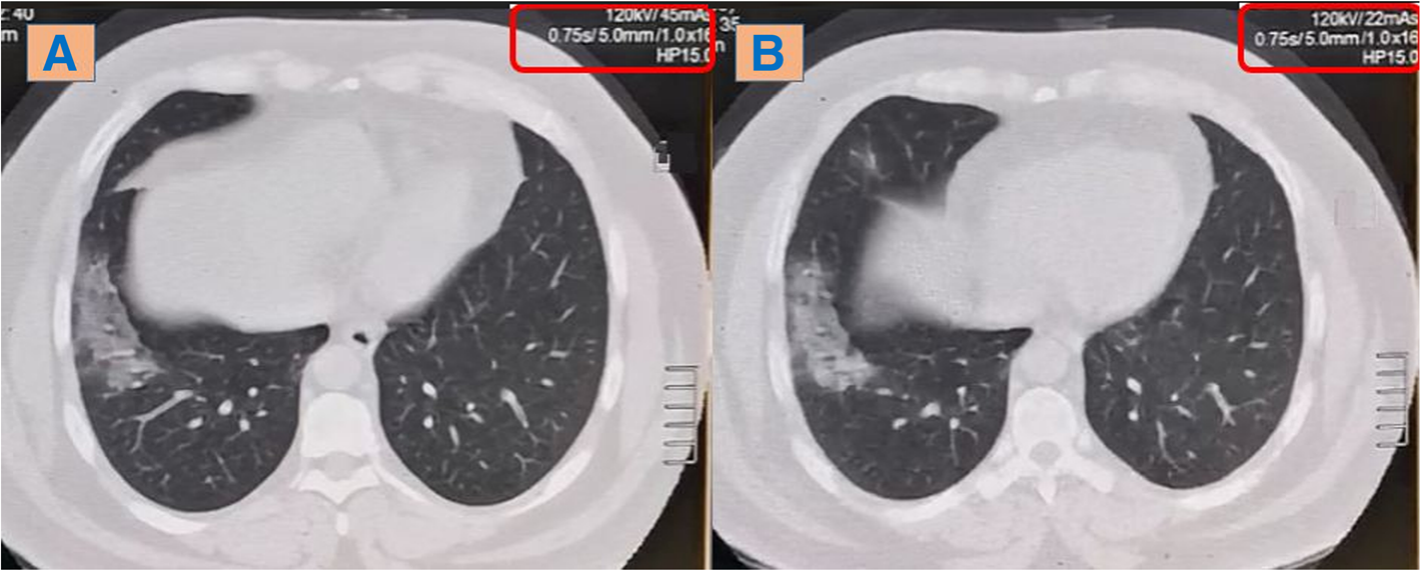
A 83-kg male patient complaining from fever and cough; axial CT chest lung window showing right lower lobar single ground-glass patch. a Low-dose CT. b Ultra-low-dose CT
